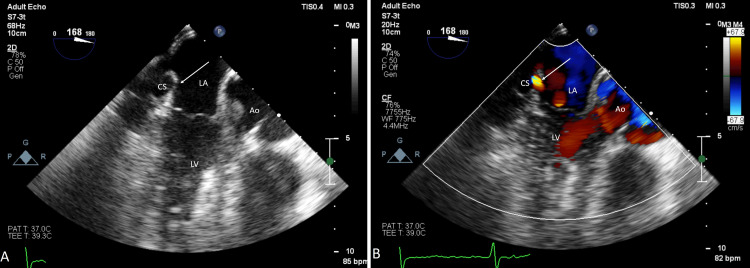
2D transesophageal echocardiographic images: (A) modified view showing the CS. White arrow showing defect on the roof of the CS; (B) view with color Doppler. White arrow shows color flow across the defect on the roof of the CS. 2D: two-dimensional, Ao: aorta, CS: coronary sinus, LA: left atrium, LV: left ventricle.
